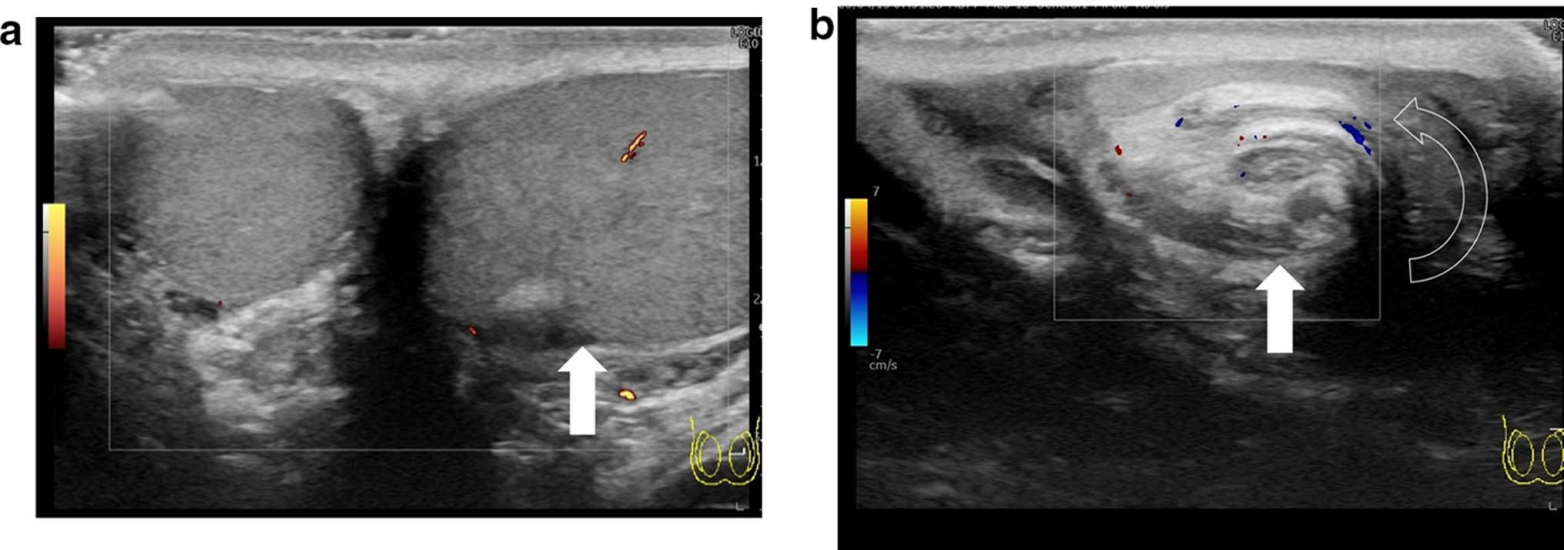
Ultrasonographic images of a 14-year-old boy with left testicular torsion. a The left testis is swollen, and the axis of the left testis is abnormal compared with that of the right testis. b The whirlpool sign is evident. Movie before manual detorsion; ultrasonogram shows the inward twisting of the left spermatic cord (curved arrow). The spermatic cord shows 360° twisting. Manual detorsion is performed via outer direction based on this finding. Movie after manual detorsion; ultrasonogram shows that the twisting of the spermatic cord is resolved, and redundant spermatic cord (arrow) is evident. A pseudomass (arrowhead) composed of congested epididymis, proximal vas deferens, and redundant vascular bundle is visualized after successful manual detorsion
